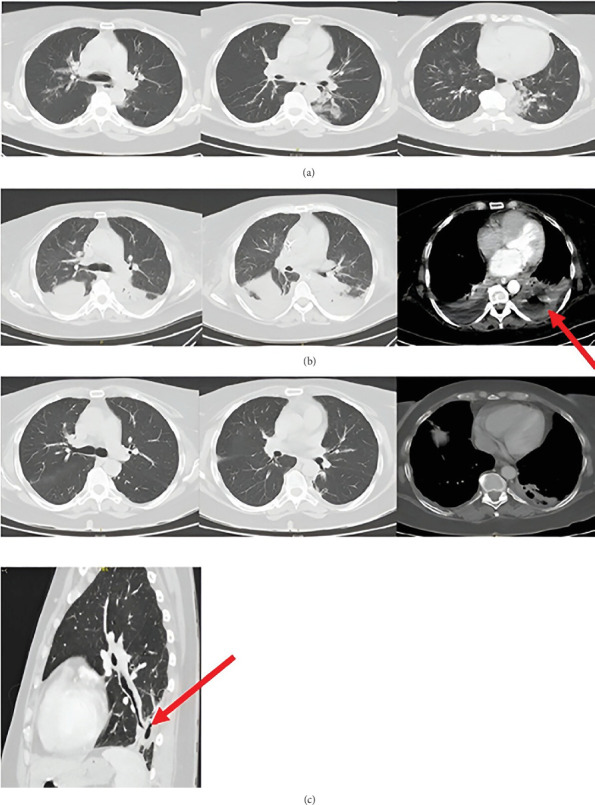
(a) CT scans performed on admission on the 14th of March 2024. Follow-up CT scans performed on the (b) 5th of April 2024 and (c) 29th of May 2024—Case 3. (b, c) The abscess is marked with an arrow.
